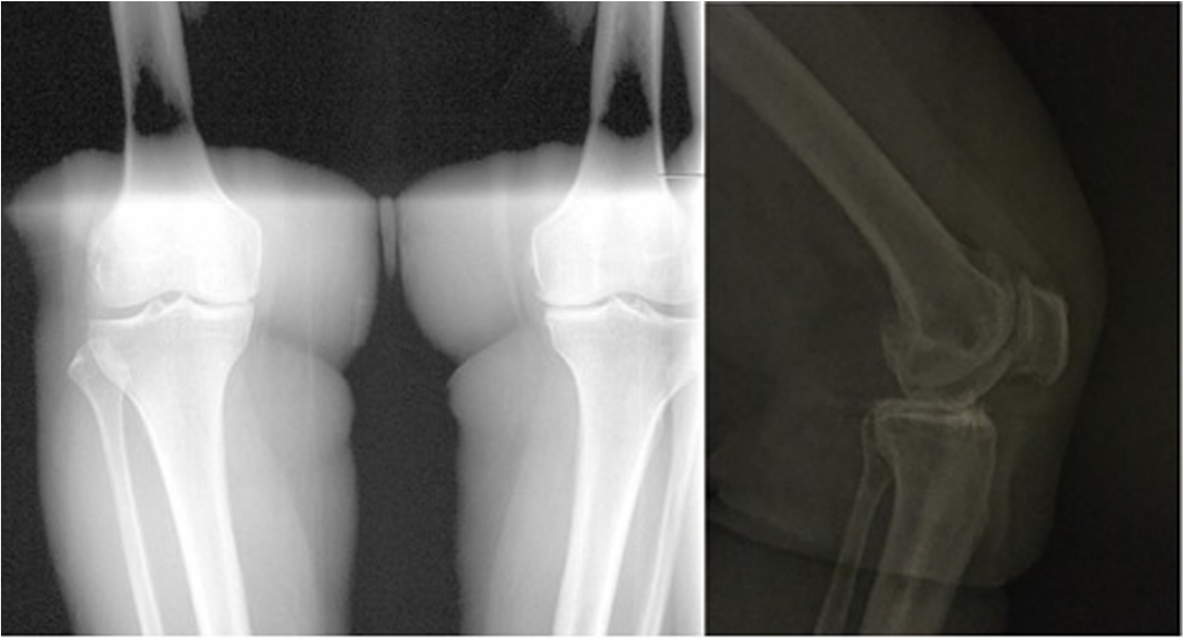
Preoperative AP and lateral X-ray of the same patient
